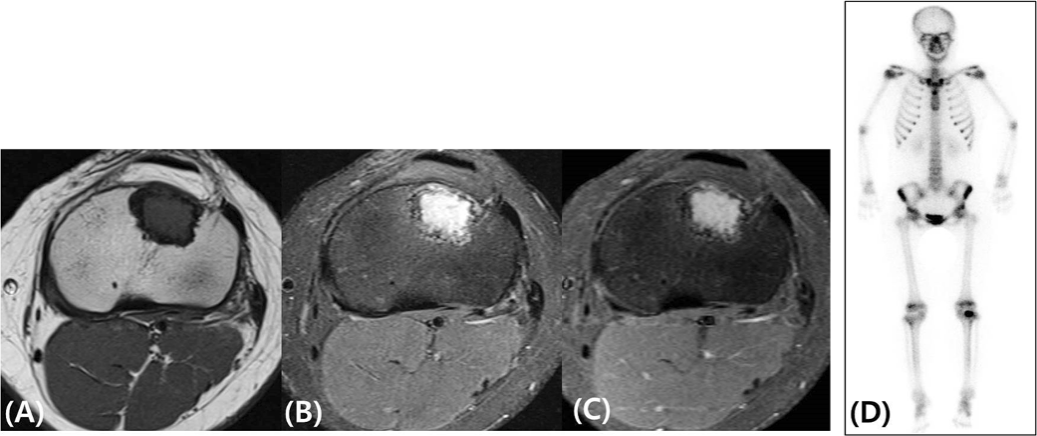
Magnetic resonance imaging and scintigraphic images. Magnetic resonance imaging scans show no peripheral bone edema and no fluid-fluid level. (A) T1-wieghted coronal magnetic resonance image (echo time/repetition time, 12/566ms) shows hypointensity of a proximal tibial lesion. (B) T2-weighted image (echo time/repetition time, 50/3660ms) shows hyperintensity. (C) Enhanced image (echo time/repetition time, 12/584ms) shows homogeneous enhancement. (D) Whole-body bone scan shows increased focal uptake only at the proximal epiphysis of the left tibia.
